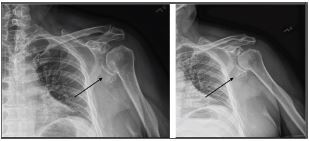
Anteroposterior (left) and oblique (right) views of the left shoulder plain radiograph, showing the inferior rim of the fractured glenoid with extension through the body displaced inferiorly (arrows). Based on the Ideberg classification system of glenoid fractures, this fracture pattern is type II.
